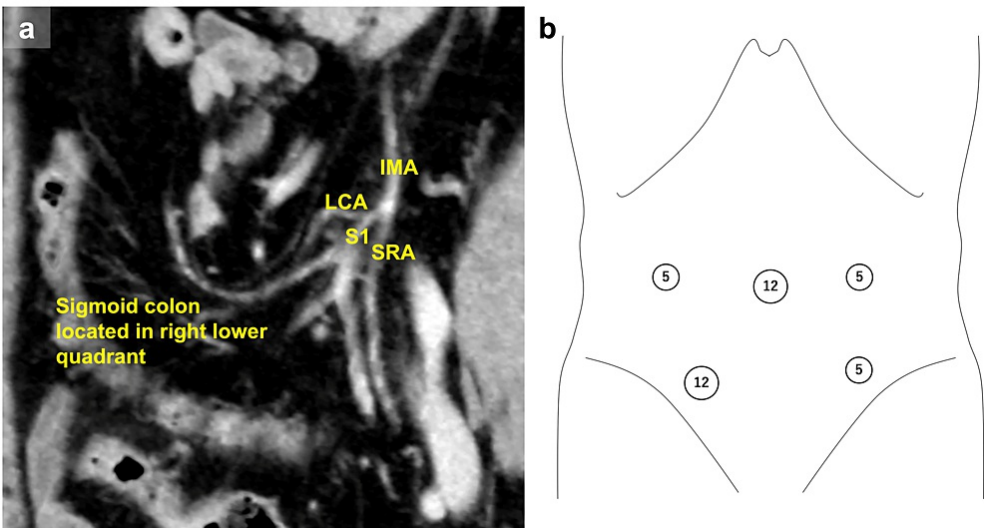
Preoperative enhanced-contrast computed tomography and port placement for laparoscopic sigmoidectomy. (a) Enhanced-contrast computed tomography revealed the sigmoid colon located in the right lower quadrant and a bear-claw inferior mesenteric artery; inferior mesenteric artery (IMA), left colic artery (LCA), first branch of the sigmoid artery (S1), and superior rectal artery (SRA). (b) Port placement for laparoscopic sigmoidectomy. The main operator switched the left side of the patient depending on the tumor location. We used the umbilical port for extracorporeal approach.
